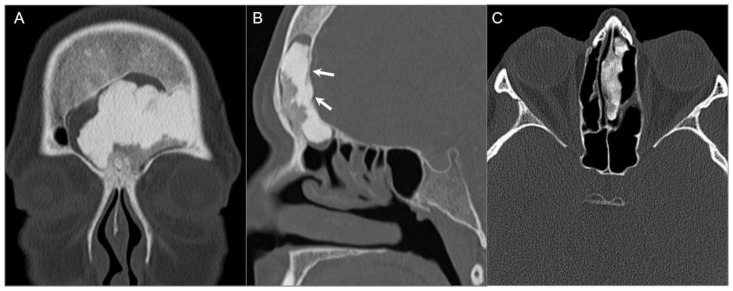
Osteomas in two different patients. (A) Coronal and (B) sagittal CT images show a large frontal sinus osteoma with mixed dense and hypodense areas consistent with cortical and cancellous components, respectively. Note mild intracranial extension (arrows). (C) Axial CT image in a different patient demonstrates a well-defined, heterogeneously hyperdense lesion centered in the left ethmoid sinus and attached to the nasal septum.
